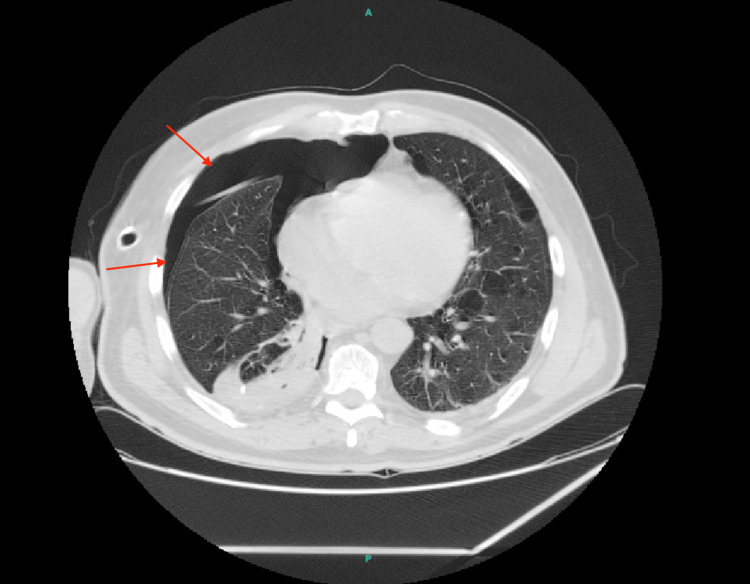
CT showing emphysematous bullae and pneumothorax with poor pleural apposition.
